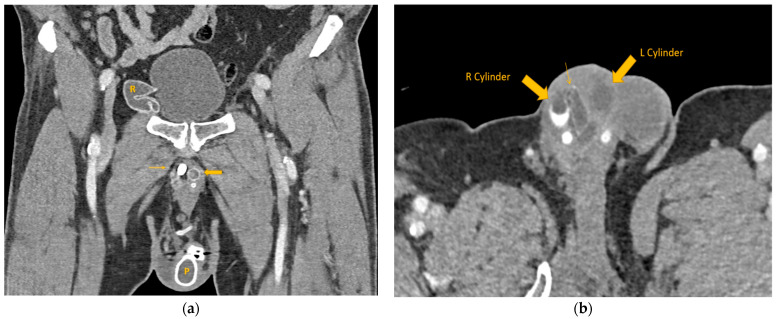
Contrast-enhanced CT of the abdomen and pelvis. (a) Coronal reformation demonstrating the decompressed reservoir in the right hemi pelvis adjacent to the bladder, and the pump noted in the left scrotum. The left fluid-filled cylinder (broad arrow) is appropriately positioned in the left corpus cavernosum. At the same level, the metallic rear tip extender is seen in the right corpus cavernosum (thin arrow) due to anterior migration of the right cylinder. (b) Axial image focused on the proximal penis, demonstrating the right and left cylinders with focal kinking of the proximal right cylinder (thin arrow) and central inferior extension of the cylinder, at the site of suspected urethral erosion. R: reservoir, P: pump.
